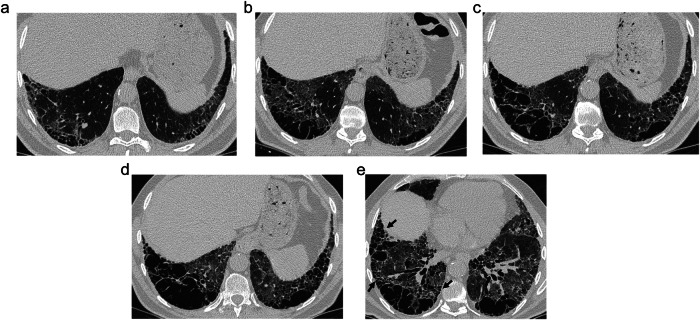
Radiological evolution of traction emphysema in a male smoker who was 59-years-old at the time of the first scan. a–d Yearly CT scan follow-up during a period of 4 years shows progression of bilateral basal subpleural cystic lesions with progressive enlargement and confluence. In e, honeycombing and traction bronchiectasis are seen in the vicinity of the traction emphysema lesion (arrows). The patient developed a severe progressive deterioration in exercise capacity
